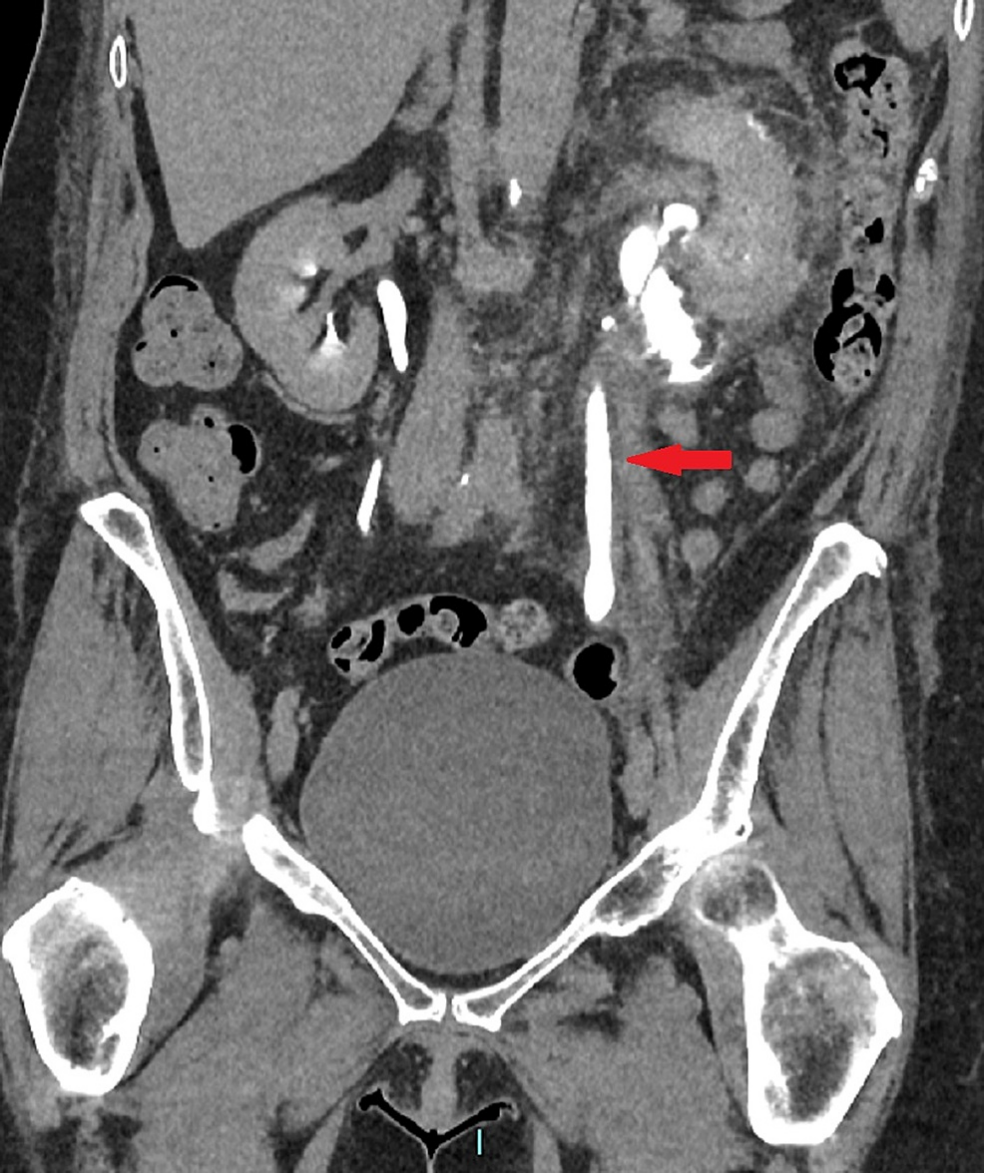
Coronal contrast CT showing mild dilatation of the left ureter in comparison with the other side one week post traumatic injury (red arrow)
